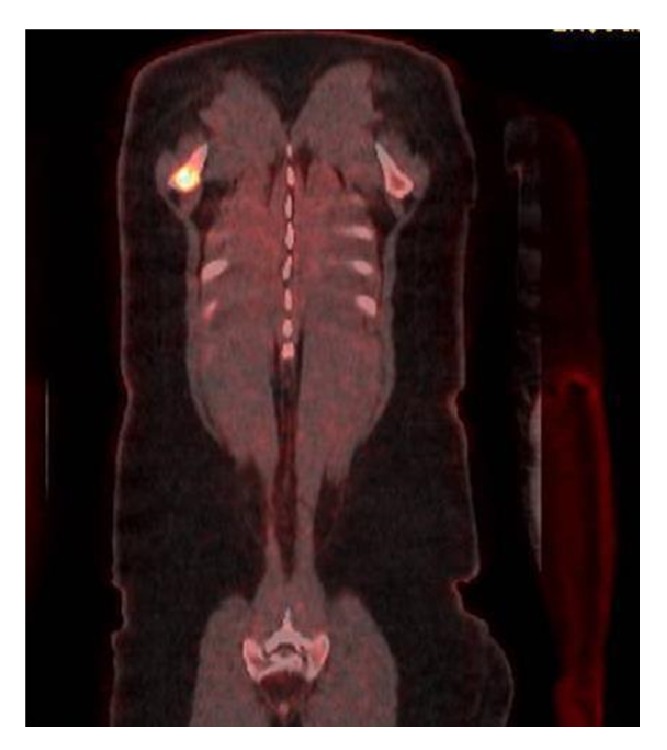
FDG avid lesion in right scapular bone.
